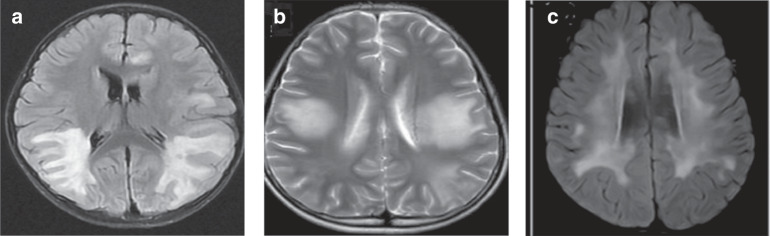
MRI features of cerebral leukodystrophy-like phenotype. a Paraventricular symmetric white matter lesions. b Large supratentorial symmetric cerebral white matter lesions. c Diffuse subcortical white matter changes.
